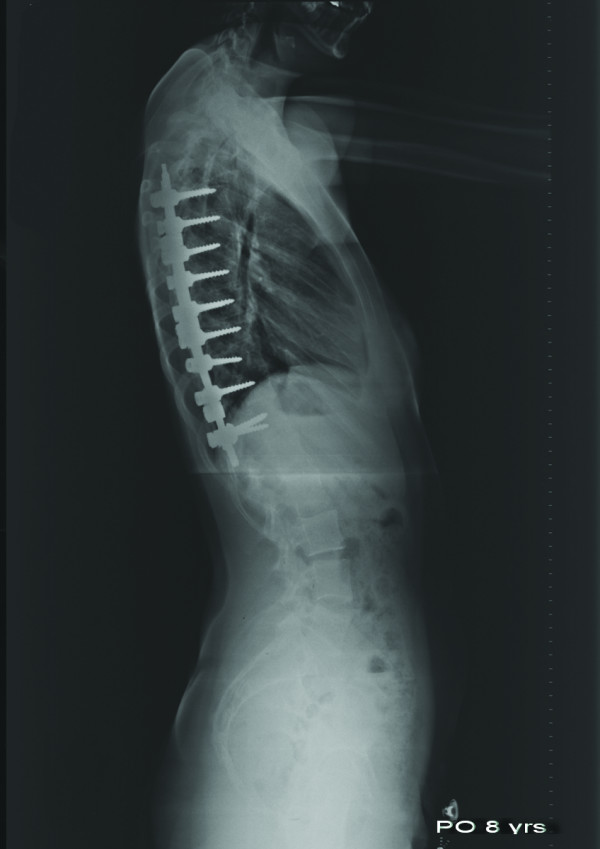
Postoperative lateral radiograph of the same patient taken 8 years after surgery. Sagittal alignment was well maintained.
